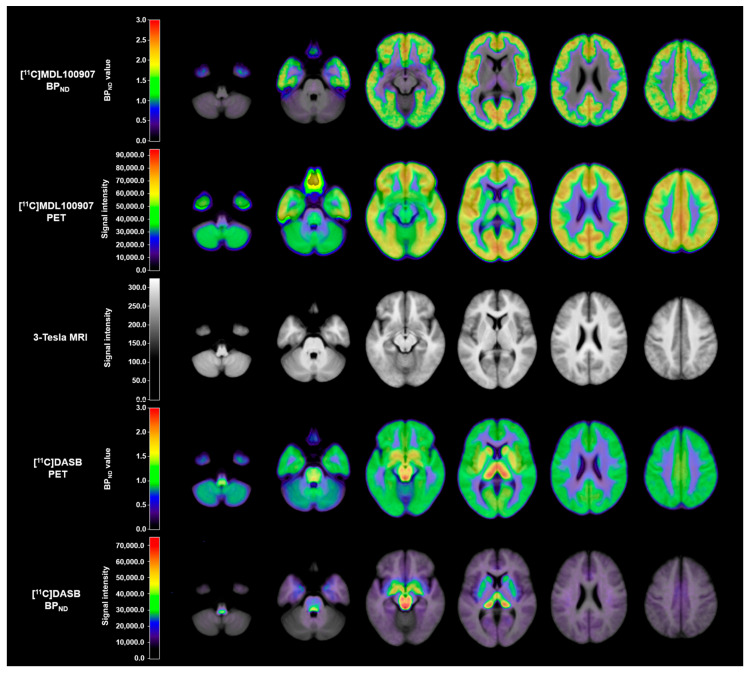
Representative average images of [^11^C]MDL100907 BP_ND_, [^11^C]MDL100907 PET, [^11^C]DASB BP_ND_, [^11^C]DASB PET, and corresponding 3-Tesla MRI in healthy individuals. BP_ND_, binding potential with respect to non-displaceable compartment; PET, positron emission tomography; MRI, magnetic resonance imaging.
